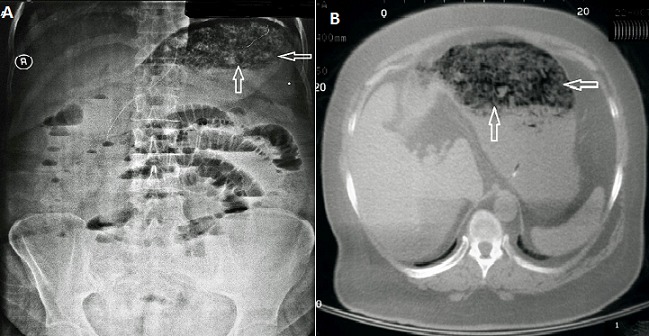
A) in the patient's direct abdominal radiographs, there was severely distended stomach and the gastric lumen had been filling with opacities; B) intenses of tissue and isodense density which fills the lumen are available in the abdominal tomography
